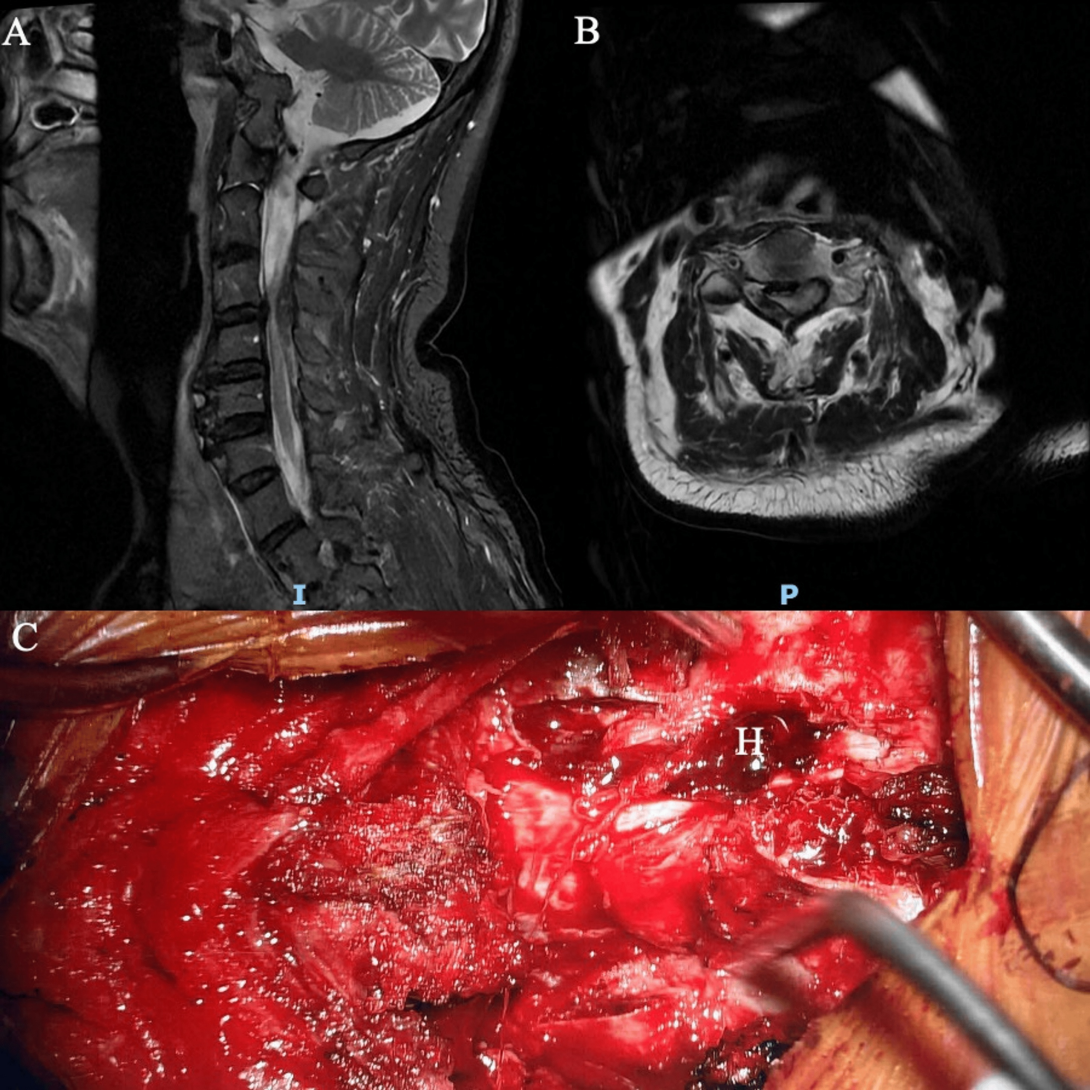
Case 2 MRI cervical spine (A) sagittal and (B) axial views showing right-sided focal epidural hematoma with cord edema spanning the C2-C4 levels. (C) Intraoperative photograph with hematoma shown (labeled “H”)
